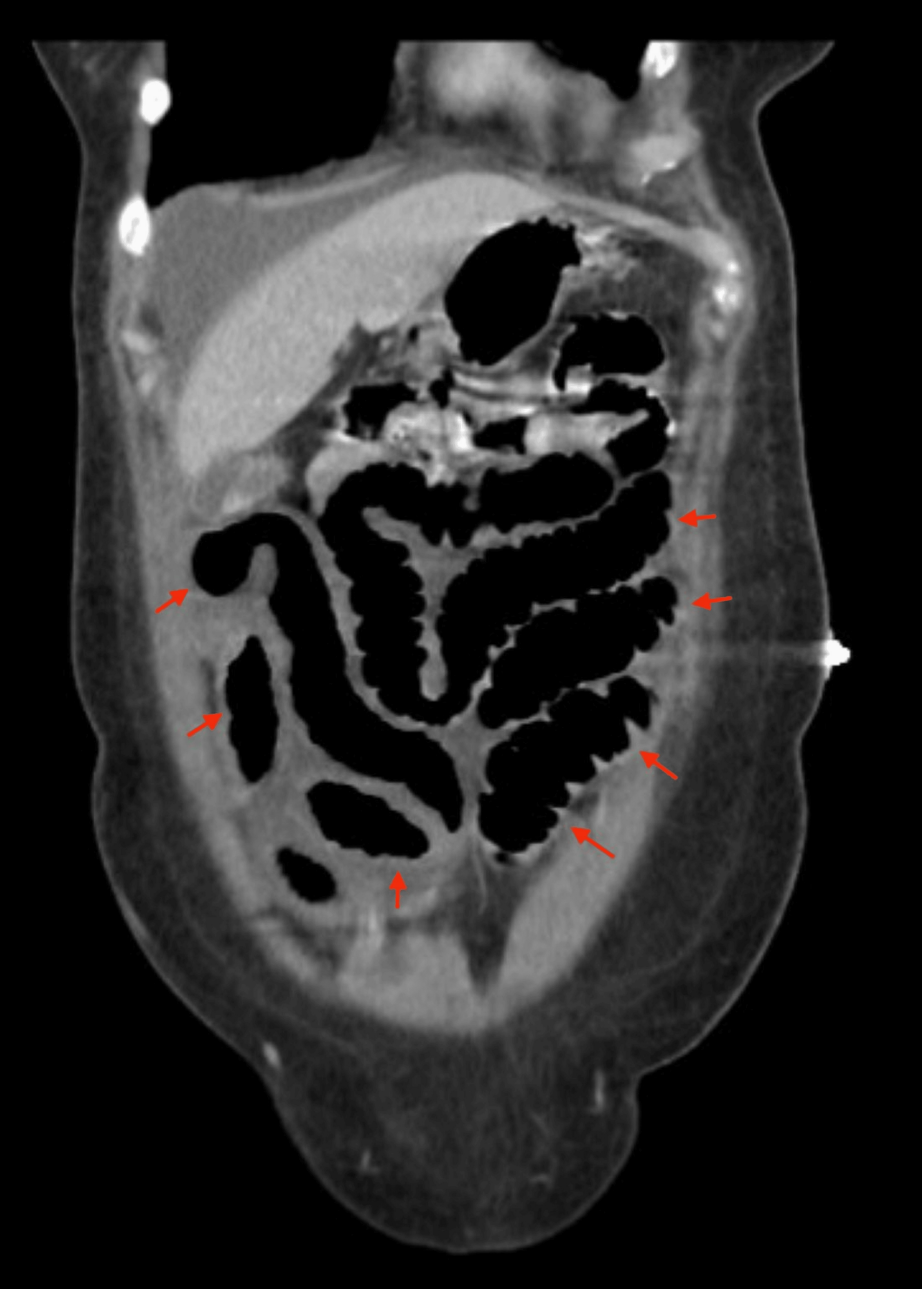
Coronal CT image demonstrating diffuse small bowel wall thickening.
